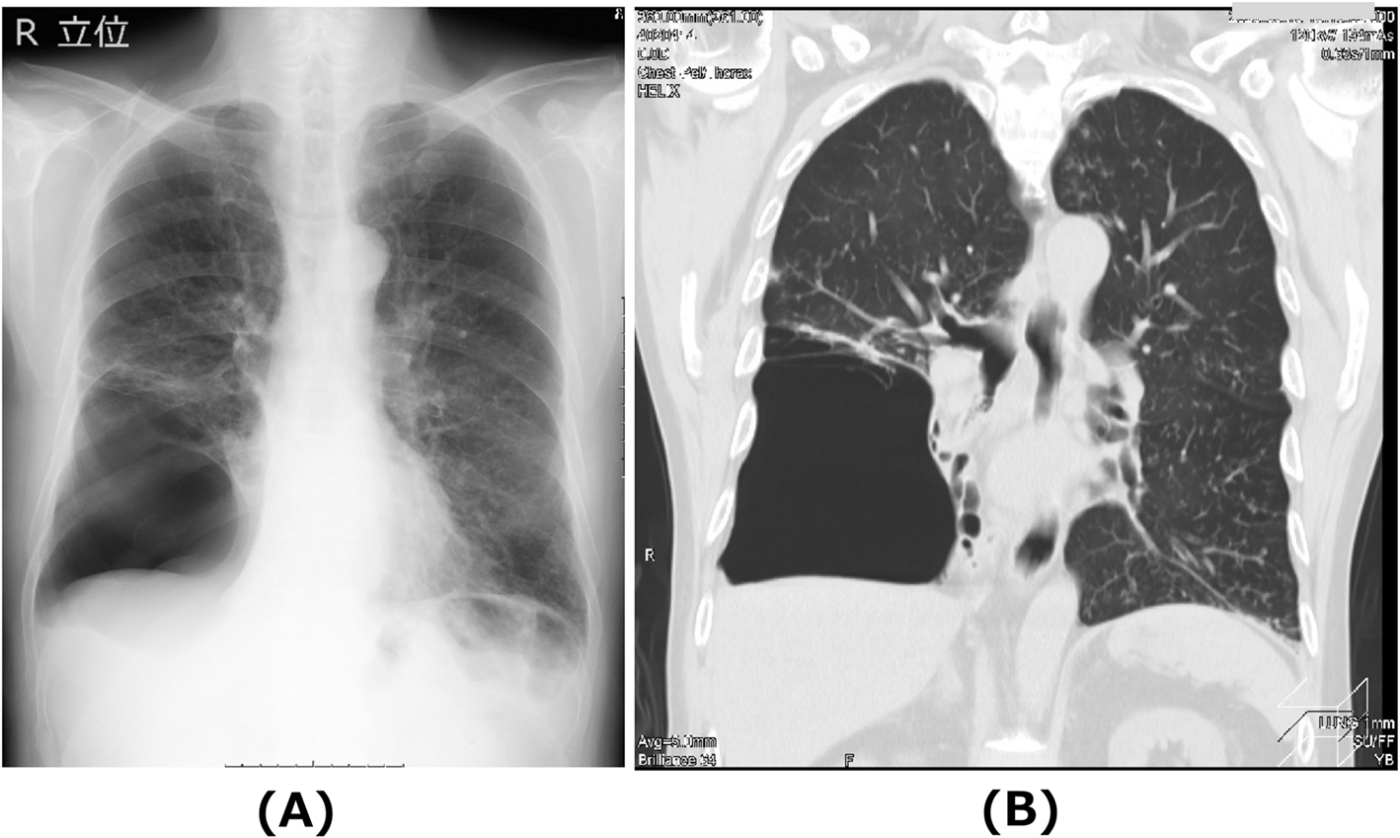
Preoperative chest radiography (A) and computed tomography (B) images. The giant bulla occupying the lower half of the right hemithorax compressed the middle and lower lobes of the right lung, leading to atelectasis. The right upper lobe had good air content
